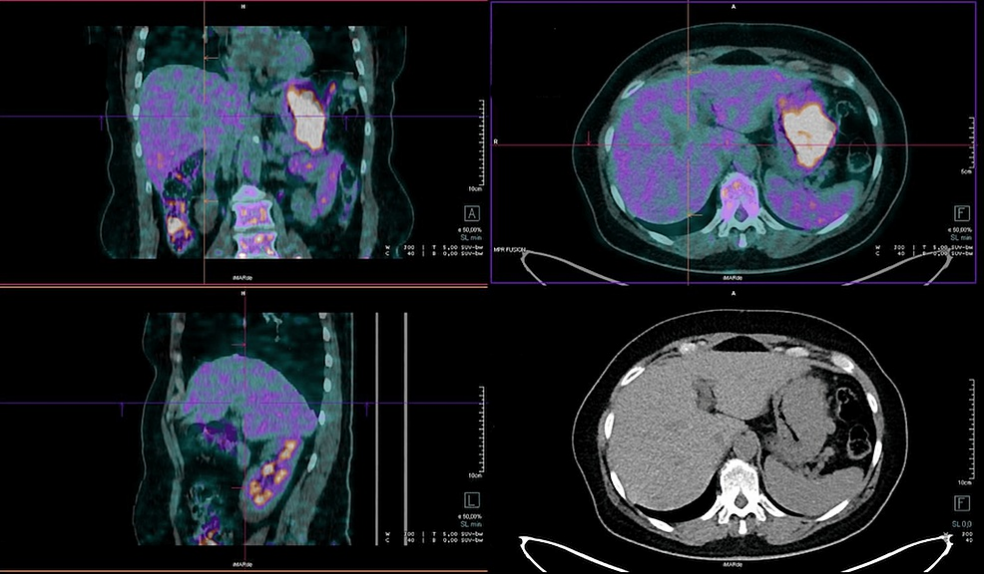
Preoperative 18-FDG PET: enhanced glucose accumulation was demonstrated on the body of the stomach, without significant radioactivity in the liver 18-FDG PET: 18-FluoroDeoxyGlucose Positron Emission Tomography
